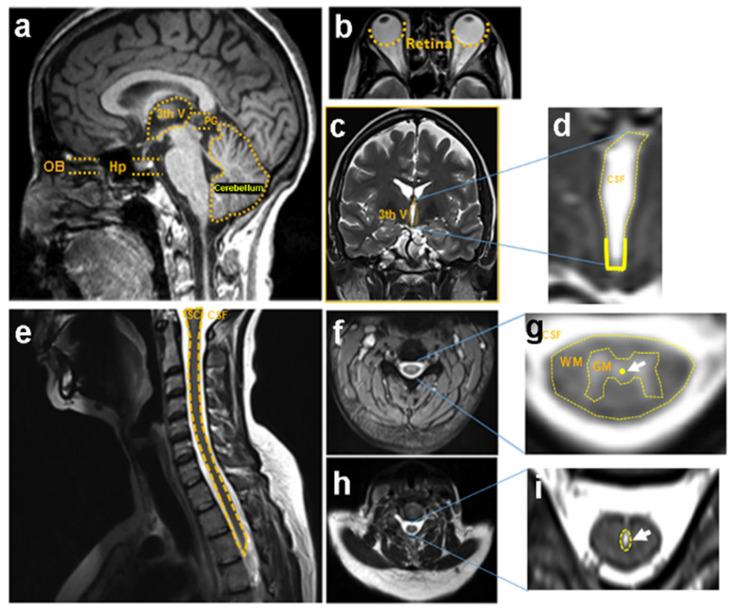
Locations of the aldynoglia cell subpopulation in the human CNS. The magnetic resonance imaging show neural niches for aldynoglia in the brain (a–d) and spinal cord (e–i): olfactory bulbs, hypophysis, third ventricle, pineal gland, cerebellum in (a), and both retinas (b). A coronal section of MRI showing the lateral ventricles (c) with enlarged third ventricle filled with cerebrospinal fluid (d), high presence of aldynoglia cells in the floor, and the lateral walls are marked. Spinal cord as a broad zone for aldynoglia, sagittal MRI section of the cervical zone of SC floating in CSF is shown (e). The axial section at C7 level (f) is enlarged showing the medullar channel with CSF surrounding white matter and grey matter, which contains aldynoglia cells (g). Arrows pointed to the location of resident aldynoglia in the ependyma of a normal individual (g) or activated aldynoglia in widened ependyma with hydromyelia in the spinal cord of patient (i). 3th V, third ventricle; C7, seventh vertebral cervical level; CSF, cerebrospinal fluid; GM, grey matter; Hp, hypophysis; MRI, magneting resonance imaging; OB, olfactory bulbs; PG, pineal gland; SC, spinal cord; WM, white matter.
